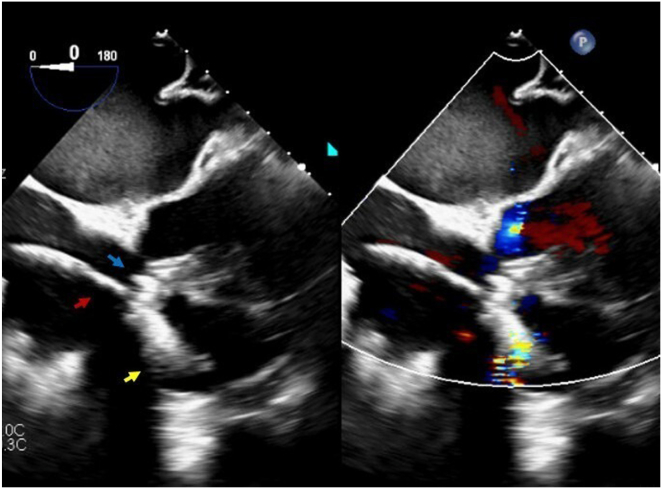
TEE mid-esophageal view at 0 degree. The *red arrow* shows the AVEIR VR delivery catheter crossing TV neo-orifice, the *blue arrow* shows TV clips, and the *yellow arrow* shows shadow from AVEIR VR inside the RV. RV = right ventricle; TEE = transesophageal echocardiography; TV = tricuspid valve.
